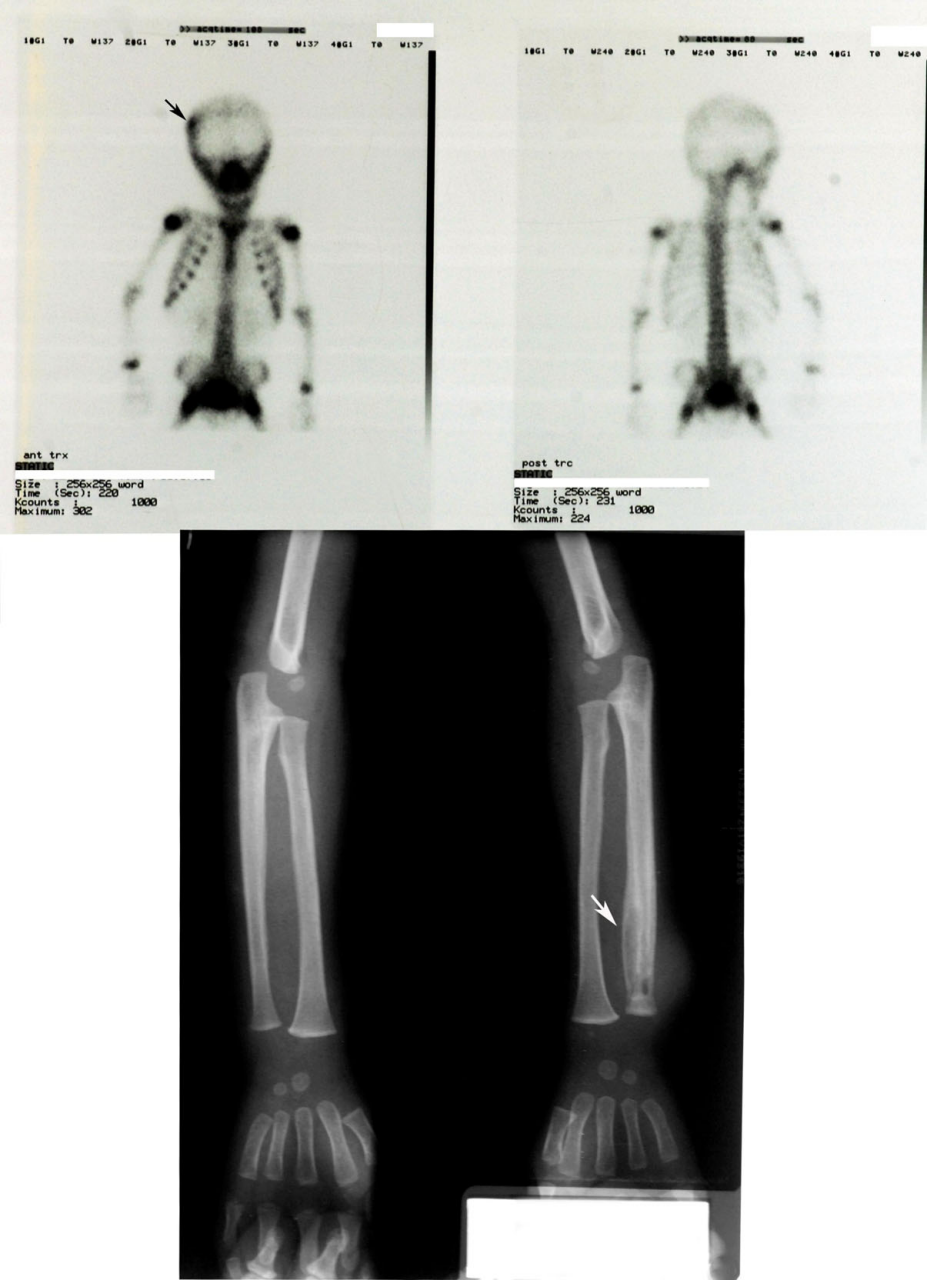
Bone Tc 99m MDP scintigraphy (a) showing increased activity on right frontoparietal bone: (b) plain radiograph of the patient showing osteolytic lesions in distal metaphyseal region of the radius.
